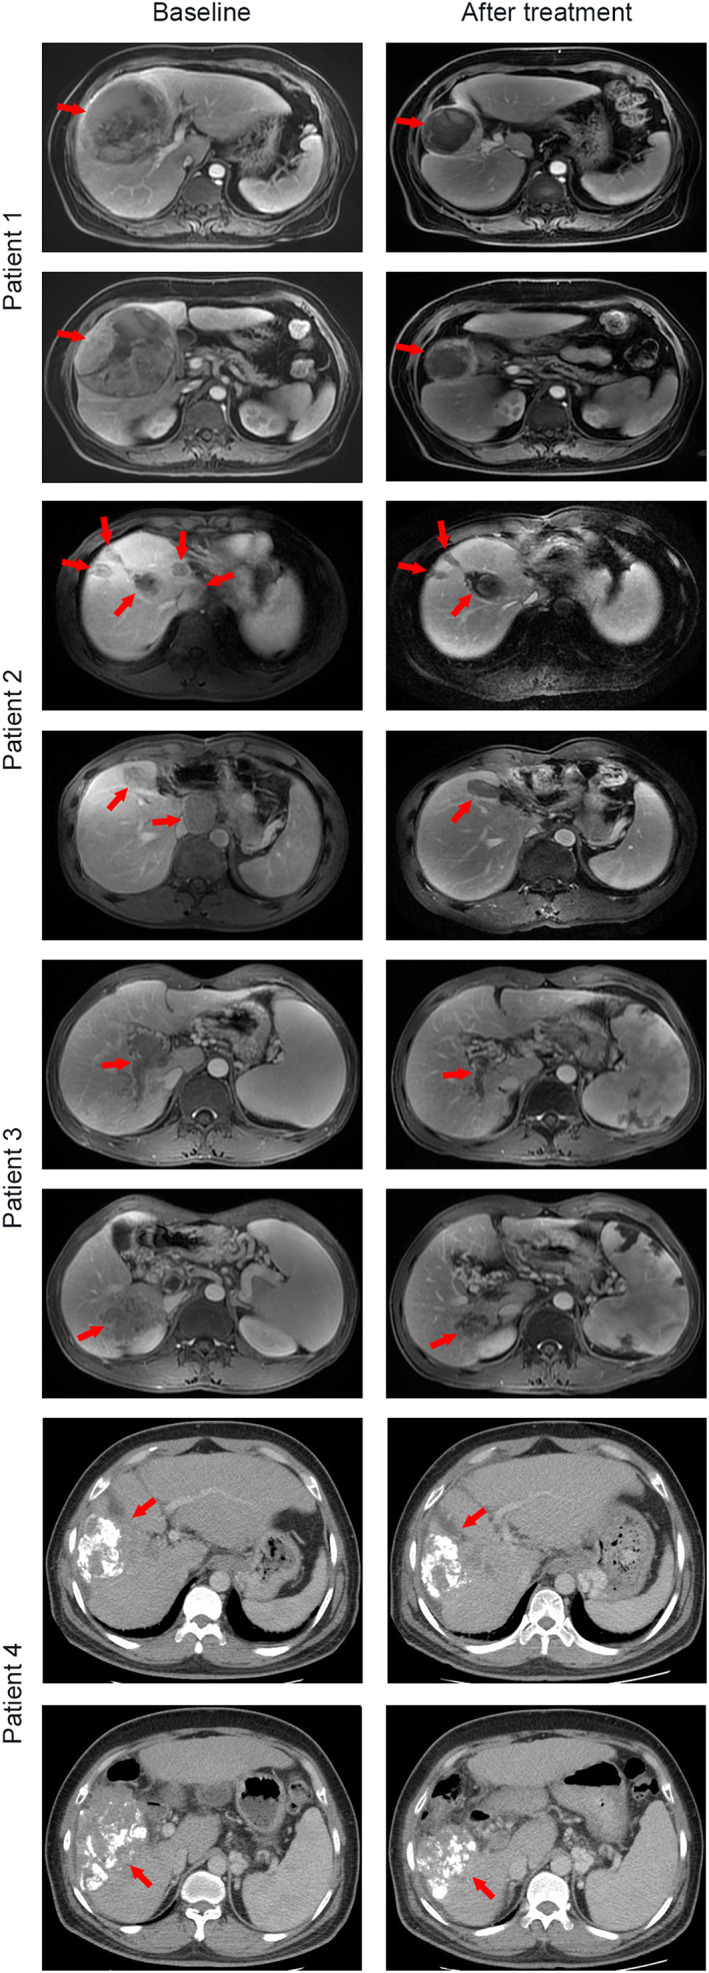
Magnetic resonance imaging (MRI) or computed tomography (CT) scans of the four responders with partial response. The four responders with partial response had more than 30% shrinkage of their tumors by MRI or CT scans. Of the four responders with their characteristics, Patient 1 had a large liver cancer with the residual liver function of less than 30% and was inoperable, Patient 2 had recurrence and metastasis after surgical treatment, Patient 3 had portal venous thrombosis, which was initially unresectable and Patient 4 had progressed after multiple interventions.
